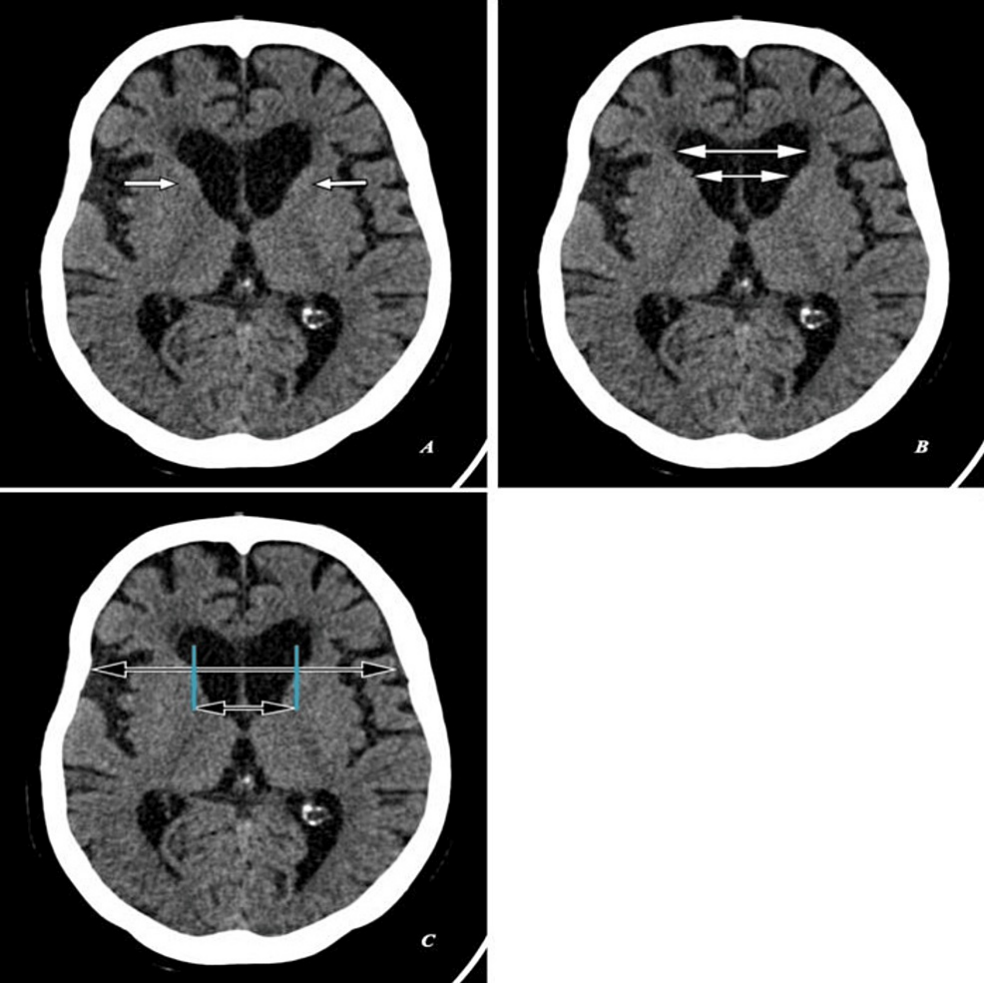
Computed tomography (CT) brain: (A,B,C) Axial sections of a 62-year-old female with unintentional, involuntary, irregular, non-patterned movements, behavioral changes, and progressive cognitive decline over the past three years. (A) Shows head of bilateral caudate nuclei mild atrophy with ex-vacuo mild dilatation of frontal horns of lateral ventricles (short white arrows). (B) Shows frontal horn width (large double white arrow) to intercaudate distance (small double white arrow) ratio (FH/CC) of 1.6, mildly decreased. (C) Shows an intercaudate distance (small double black arrow) to inner table width (large double black arrow) ratio (CC/IT) of 0.24, mildly increased.
